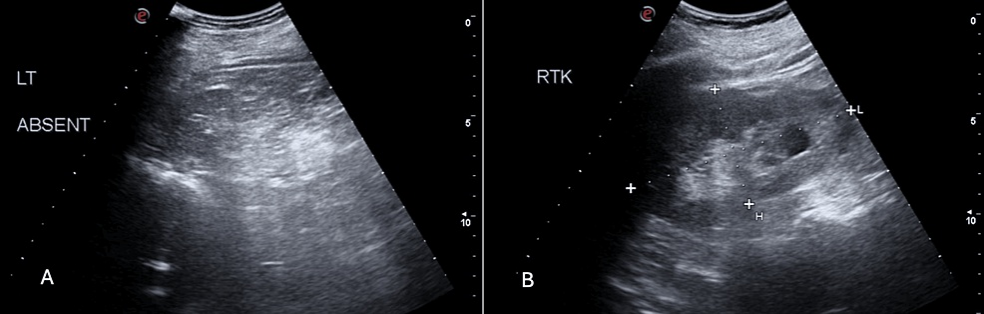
Renal ultrasound demonstrating unilateral renal agenesis. Panel A demonstrates the absence of the left kidney, indicative of unilateral renal agenesis. Panel B displays the normal appearance of the right kidney.
